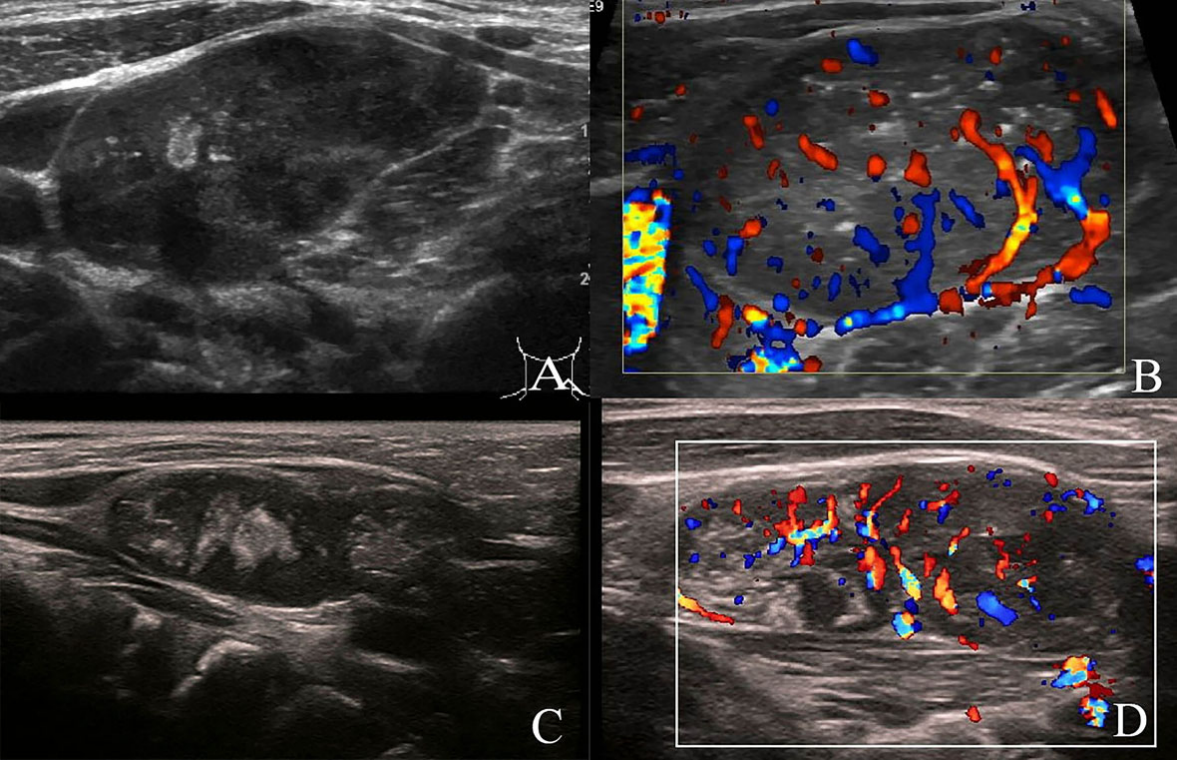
Ultrasound images of superficial soft-tissue masses due to lymphatic metastases: A, Female, 2y10m, left neck mass for four days. There were several enlarged lymph nodes in the left neck areas III and IV. The larger one was about 3.2×1.6 cm, oval in shape, with clear boundary, heterogeneous internal echo, patchy hyperechoic foci, and diffuse cortical thickening. B, Blood flow signals in enlarged lymph nodes are more abundant in the periphery than in the center. C, Female, 2y10m, left supraclavicular mass present for more than 4 months. Ultrasound image shows heterogeneous echogenic band with scattered bright echogenic spots and flaky dark area echoes. D, Color Doppler image shows hypoechoic mass with a dot-strip blood flow signal.
